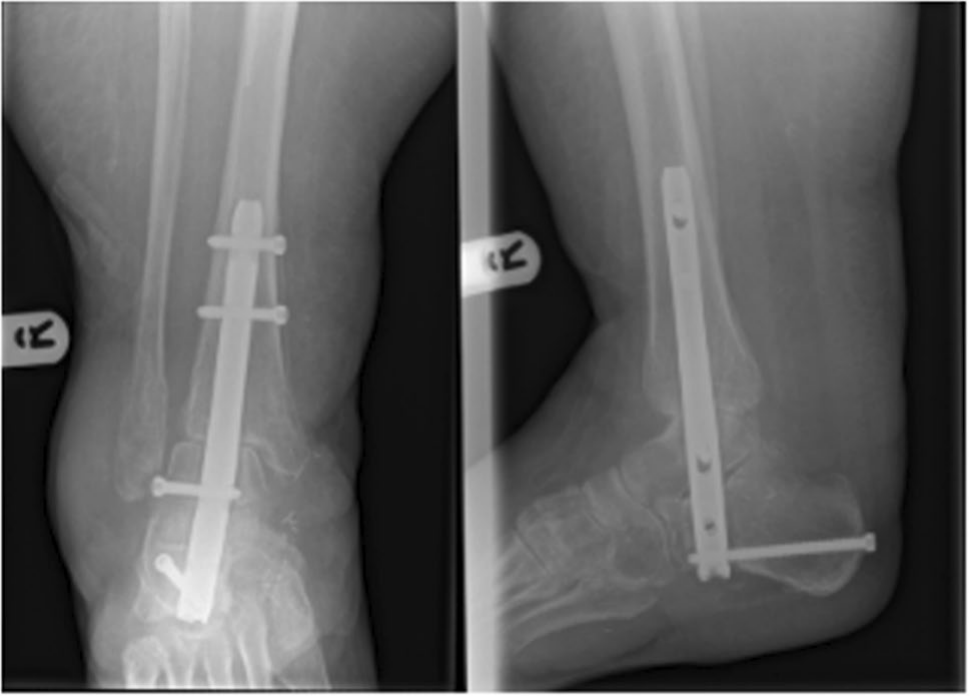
Post-operative radiographs after TCC nailing. Source: Obtained from Ref. 24
